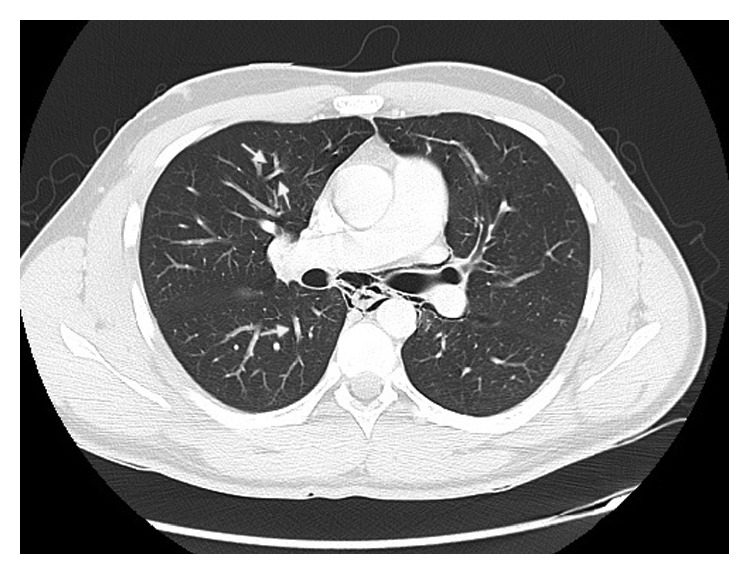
Sagittal CT showing the Macklin effect (arrows) with linear air adjacent to bronchovascular sheaths.
